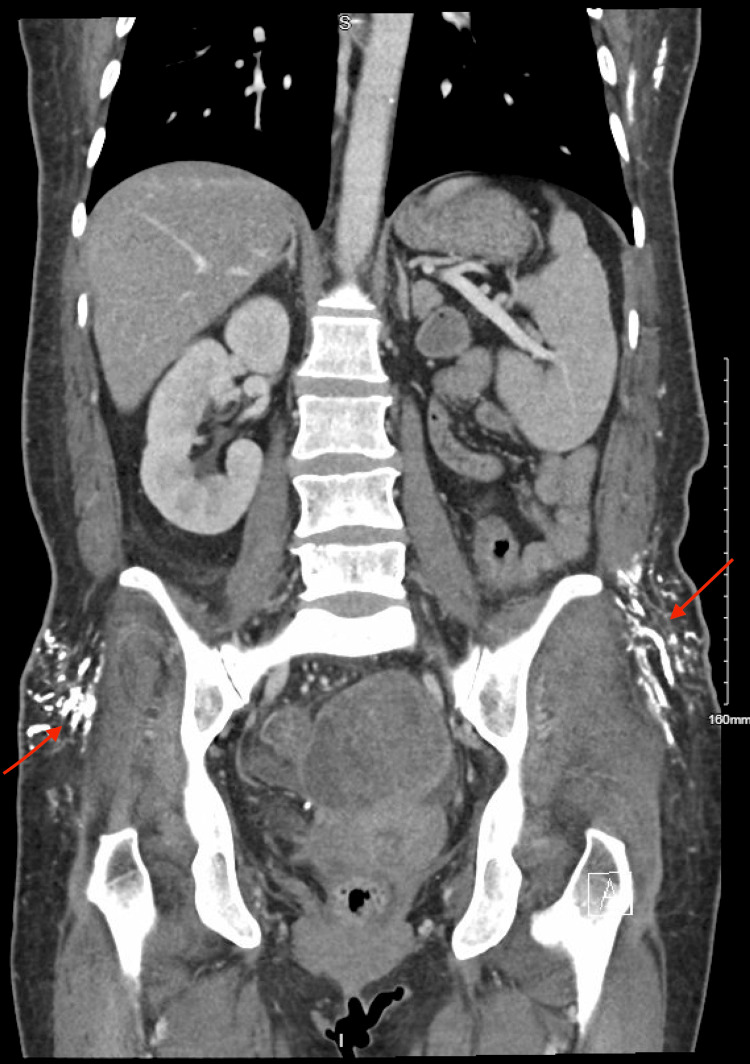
Coronal computed tomography image of the abdomen and pelvis demonstrating extensive nodular calcifications within the subcutaneous tissues of the bilateral gluteal and lateral pelvic soft tissues (arrows), consistent with extensive soft tissue calcinosis
